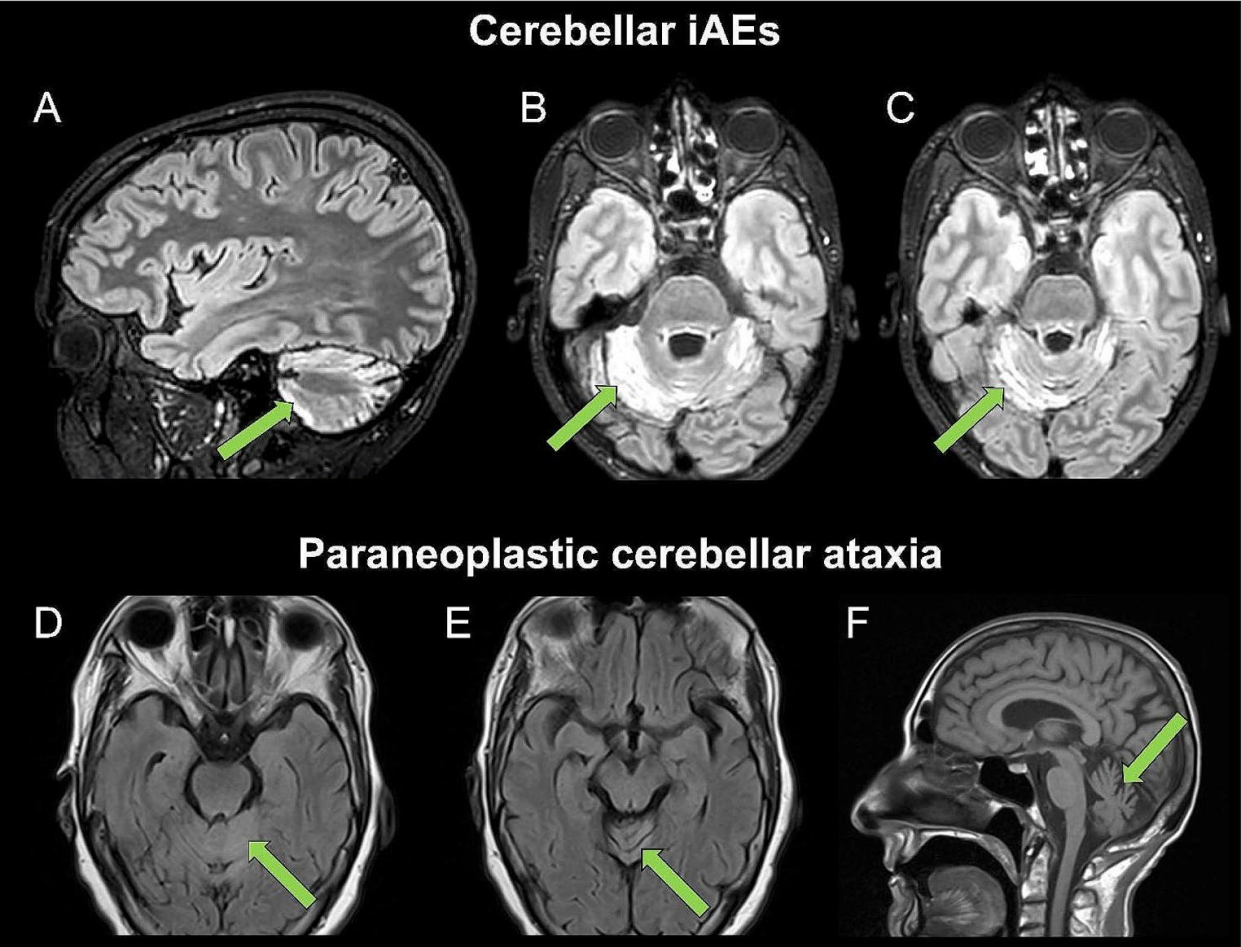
Representative brain MRI findings. Fluid attenuated inversion recovery (FLAIR) brain magnetic resonance imaging (MRI) findings in patients with cerebellar immune-related adverse events (irAEs) due to immune checkpoint inhibitors cancer treatment (A, sagittal section; B and C, axial sections) showing cerebellar hyperintensities. Brain MRI findings in paraneoplastic cerebella ataxia demonstrating mild cerebellar hypersignal (D and E, axial sections) as well as atrophy (F, sagittal section)
